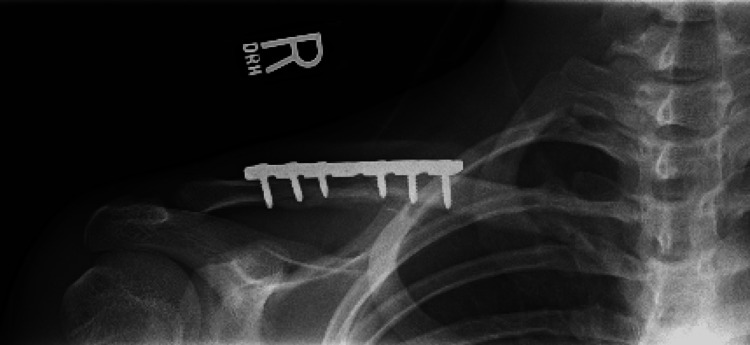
X-ray of the patient’s right clavicle (September 2010) showing the clavicle fixation screws in the patient’s right shoulder
